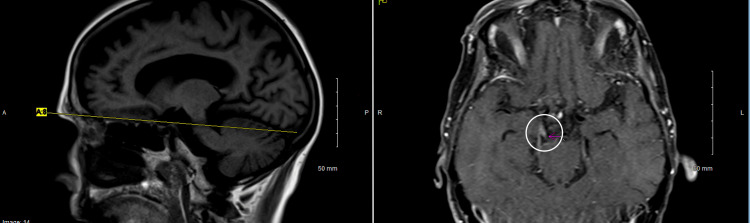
Axial post-contrast orbital MRI showing linear enhancement anterior to the superior pons and midbrain junction to the right of midline representing enhancement of the cisternal segment of the oculomotor nerve.
